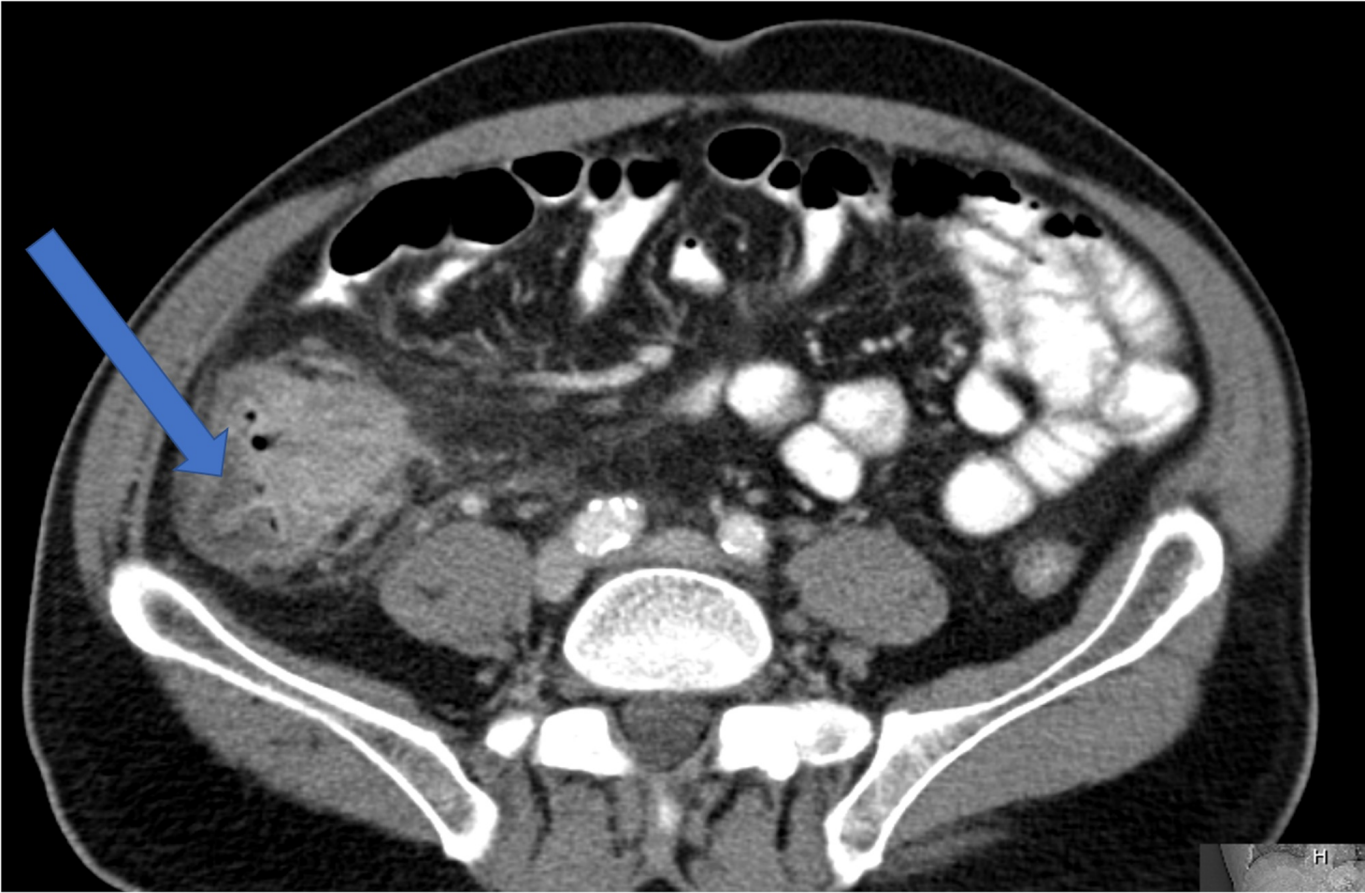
CT abdomen/pelvis scan on initial admission Mass (arrow) causing obstruction, possibly in the cecum or distal ileum
